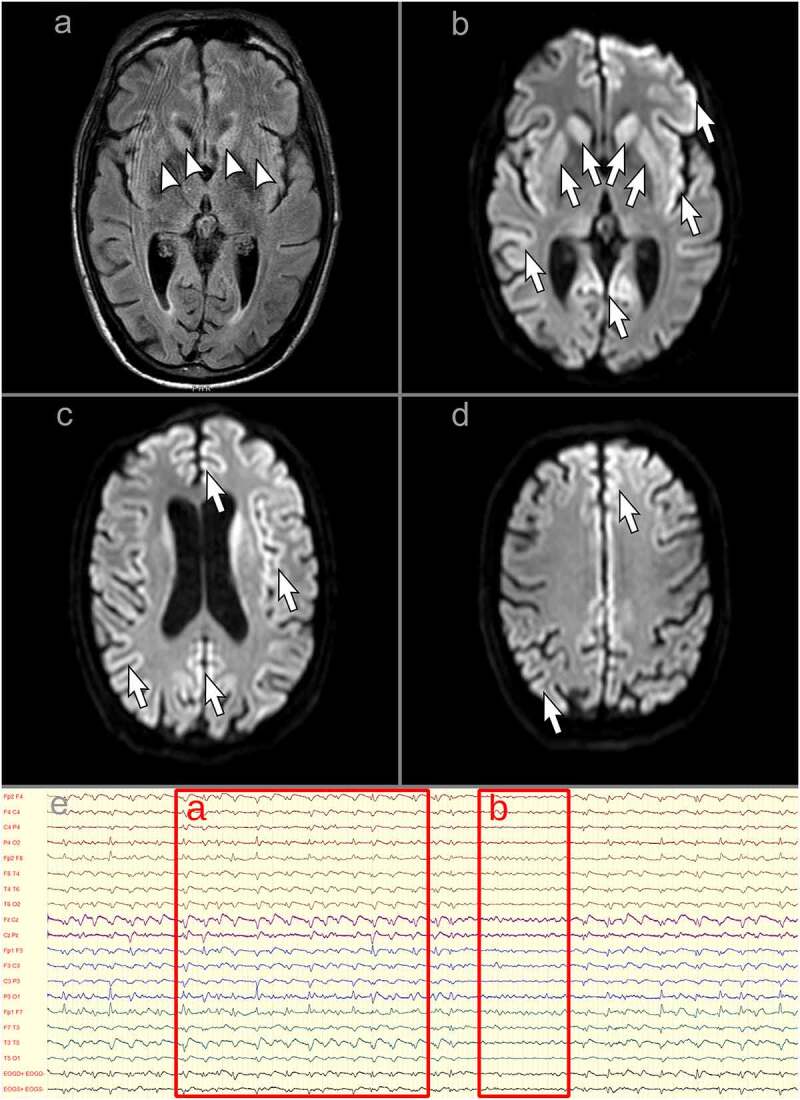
A-D: magnetic resonance imaging of the brain showing typical features of Creutzfeldt-Jakob disease: hyperintensity of the caudate nuclei and putamina on fluid-attenuated inversion recovery imaging (panel a, arrowheads) and diffusion restriction within the bilateral striatum, right fronto-temporo-insular cortex, left fronto-parietal cortex and bilateral occipital cortex on diffusion-weighted imaging (panels b-d, arrows). e: electroencephalographic recording showing typical features of Creutzfeldt-Jakob disease. a longitudinal montage is depicted. Subcontinuous generalized triphasic periodic sharp-wave complexes can be seen on this segment, with a 1–2 Hz discharge rate (a); during the short interruptions in periodic sharp-wave complex firing, a diffusely slowed background activity in the theta range can be observed (b).
